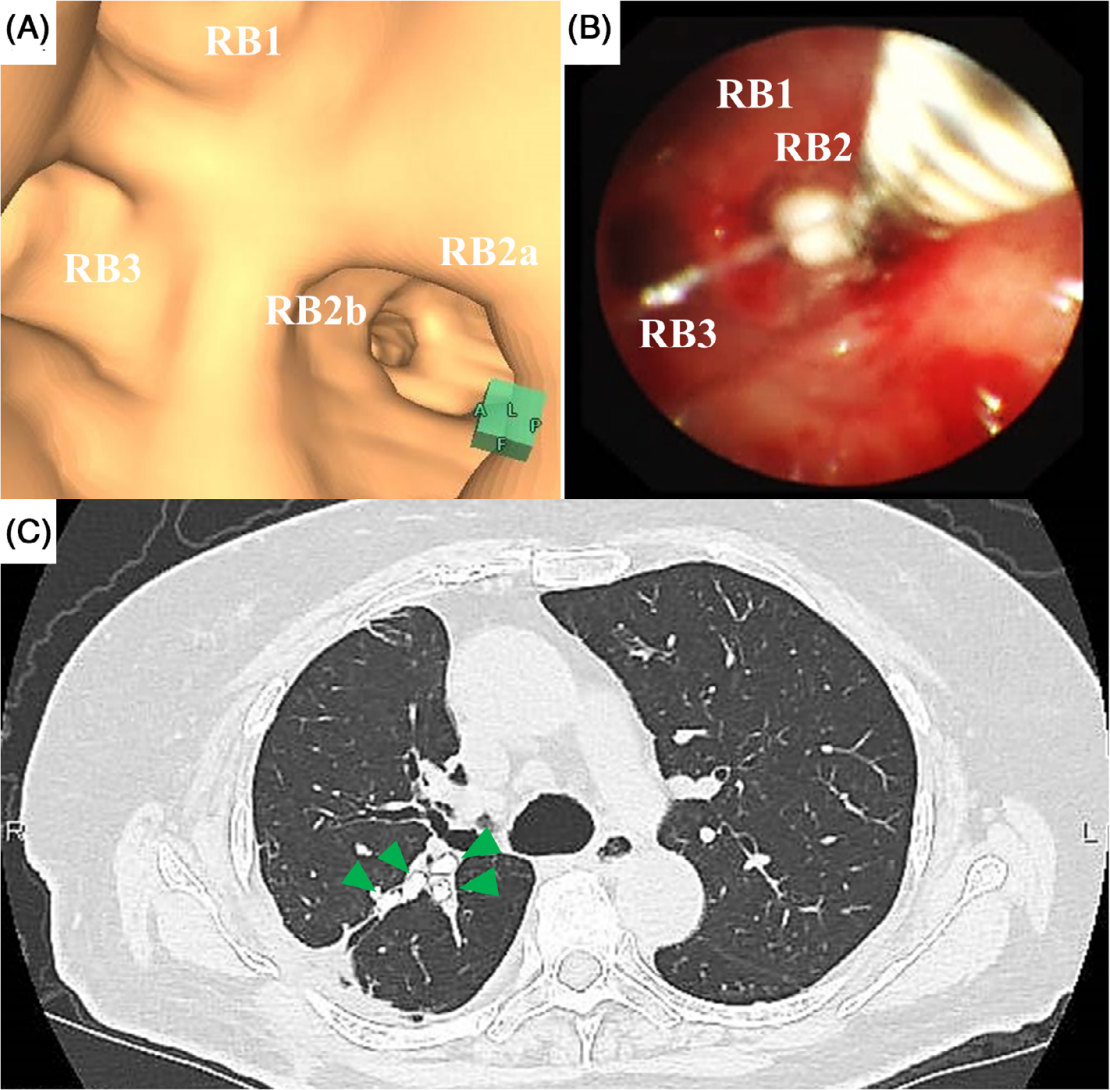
(A) Image by virtual bronchoscopic navigation of affected subsegmental bronchi. RB2b and RB2a were responsible for bronchosubcutaneous fistulae. (B) Bronchoscopic image showing insertion of an endobronchial Watanabe spigot (EWS) into RB2b. (C) Computed tomography image of the chest after the bronchoscopic procedure showed appropriate deployment of EWSs (two at RB2b, one at RB2a, and one at the root of RB2) (green arrows) and complete disappearance of subcutaneous emphysema.
